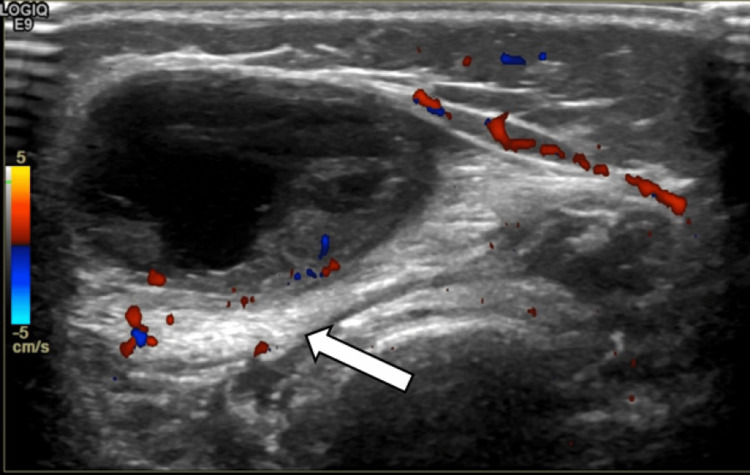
Ultrasound of the right thigh showing an intramuscular heterogenous ovoid cystic mass with septation and internal soft tissue, measuring 41 mm × 13 mm × 24 mm. Surrounding tissue exhibits increased vascularity, however, the mass itself does not appear to have internal vascularity. There is evidence of posterior acoustic enhancement (arrow).
